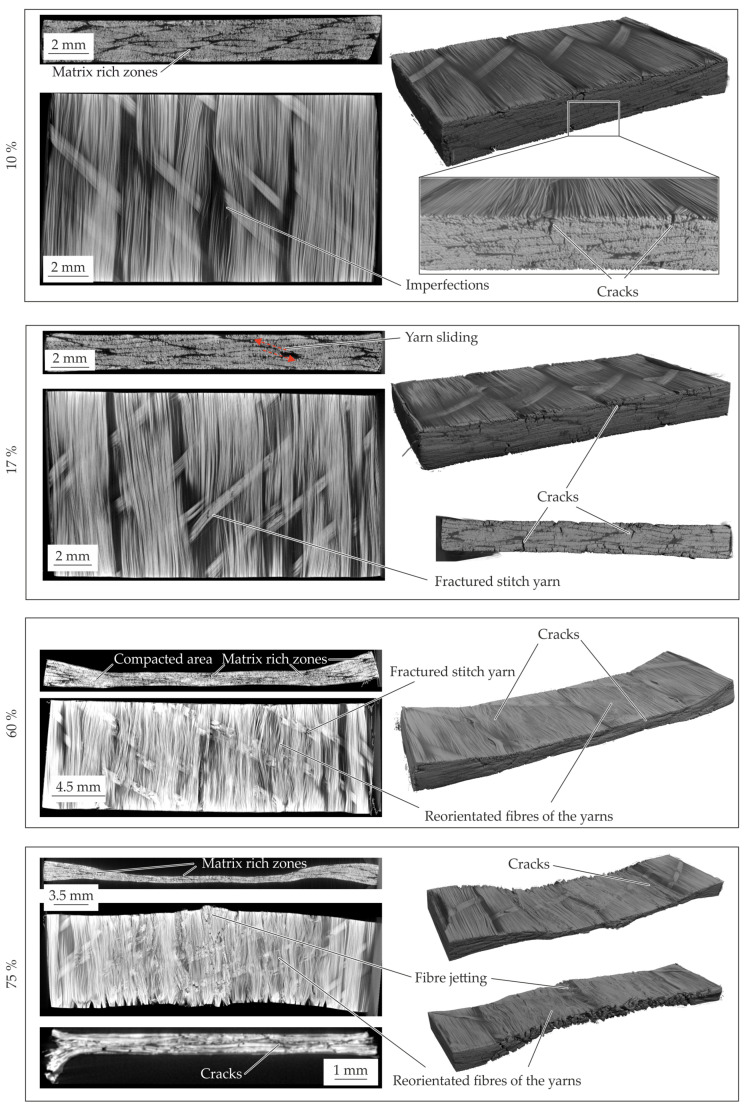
CT analysis of the 2 mm–configuration for the different compaction levels at 180 °C.
